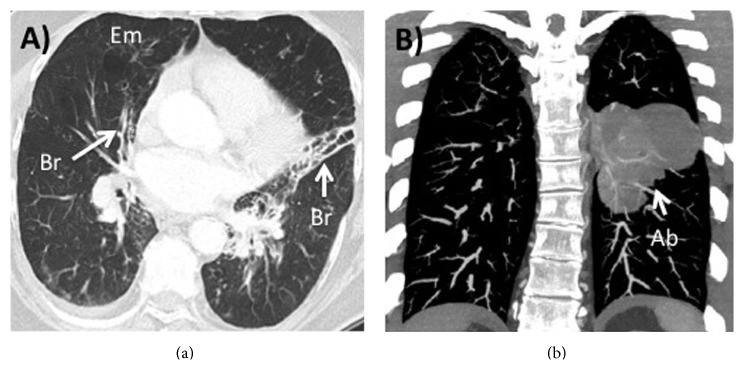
Clinical situations with NTHi infection. (a) shows a computed tomography (CT) scan of a subject with severe chronic obstructive pulmonary disease and the prescence of bronchiectasis (Br) and emphysema (Em). This patient had chronic NTHi airway colonization and exacerbations for a number of years. (b) shows a CT scan of a patient with Hyper IgE syndrome with a lung abscess (Ab) from which* H. influenzae* was cultured.
